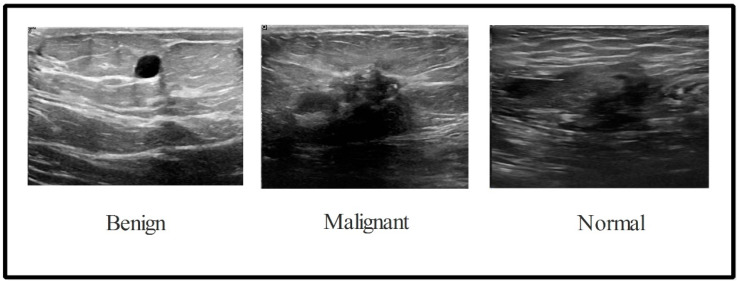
Sample ultrasound images of the BUSI dataset [59].
